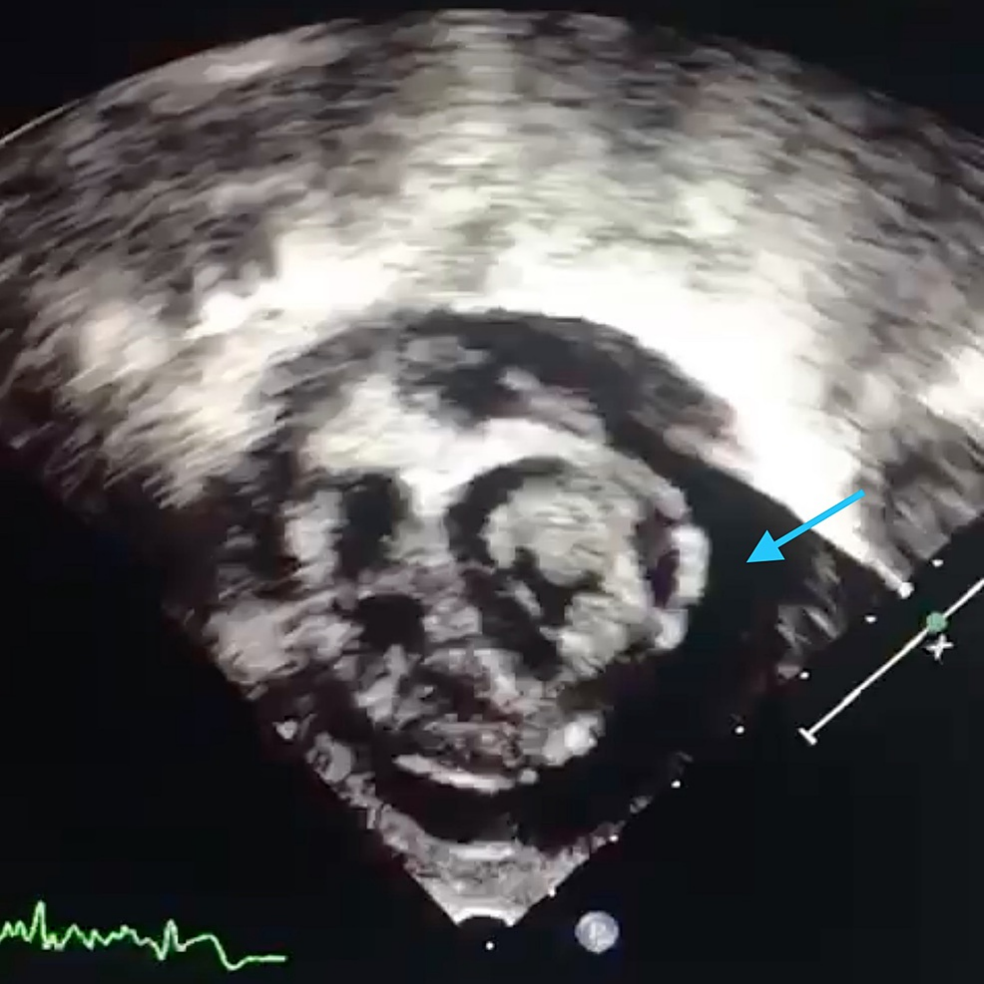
Echocardiogram subcostal short axis at the level of the papillary muscle showing pericardial effusion.
